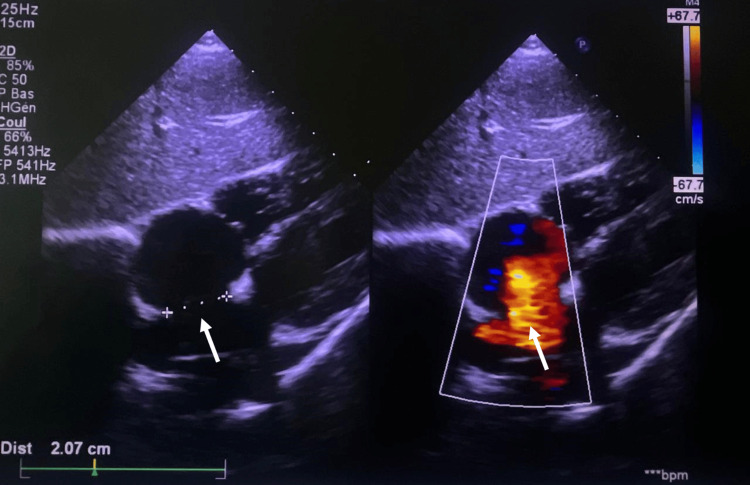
Transthoracic echocardiography (apical four-chamber view) of Case 1. The left panel shows a 2D image of the ostium secundum atrial septal defect measuring 20 mm (white arrow), with adequate septal rims. The right panel demonstrates color Doppler imaging confirming a significant left-to-right shunt (white arrow), consistent with a Qp/Qs ratio of 2.0 and systolic pulmonary artery pressure (SPAP) of 35 mmHg, supporting the decision for percutaneous closure.
